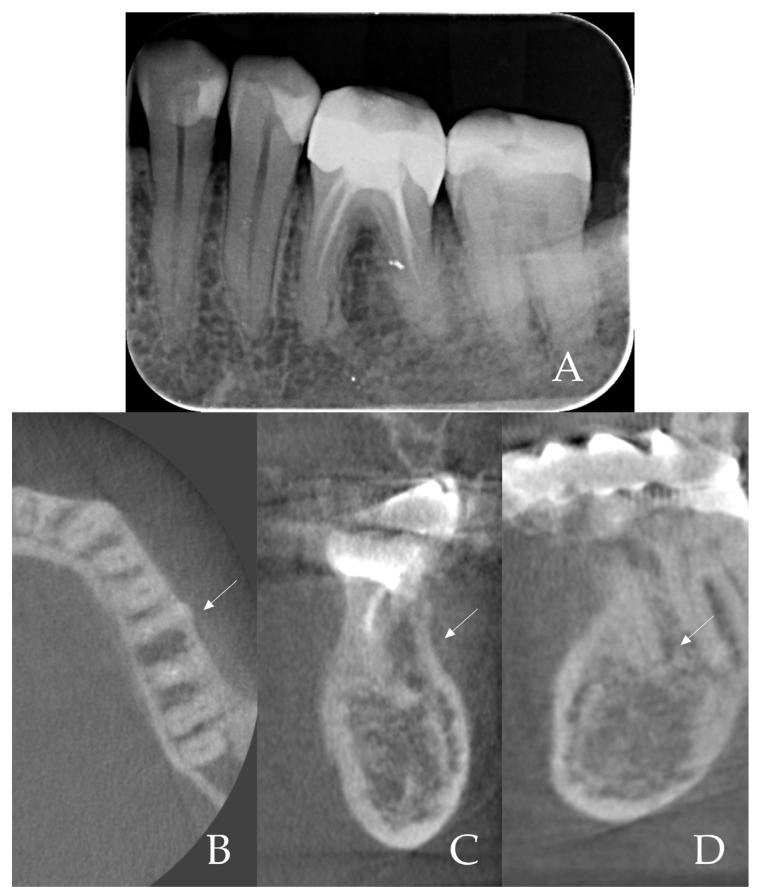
Radiographic preoperative examination. (A)—Periapical radiograph of tooth 36, indicating periapical bone destruction around M and D roots; (B,C)—preoperative CBCT view, indicating an intact buccal cortical plate marked by white arrows; (D)—CBCT view, white arrow indicating bone destruction around distal root apex.
